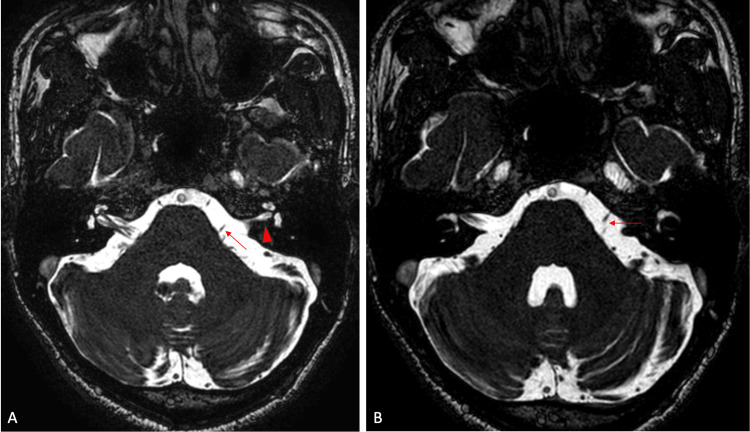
3D CISS sequence MRI images in axial plane at the level of pons: (A) hypoplastic left IAC (red arrowhead) and a hypoplastic left facial nerve arising from the lateral pons (red arrow) and (B) the hypoplastic left facial nerve coursing antero-superior to the left IAC (red arrow) 3D: three dimensional, CISS: constructive interference in steady state, MRI: magnetic resonance imaging, IAC: internal auditory canal
